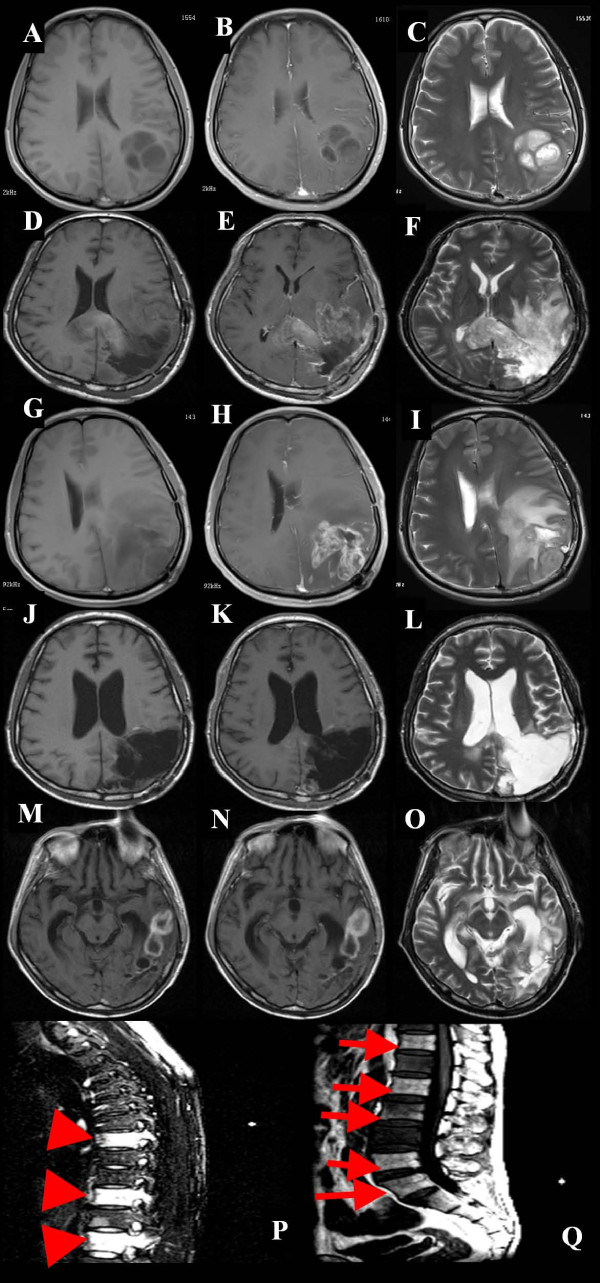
Representative axial MR images with gadolinium, taken on initial admission. (A) T_1_-weighted MR image (T_1_WI); (B) Contrast-enhanced T_1_WI and (C) T_2_-weighted MR image (T_2_WI) showing a left occipital tumor with mild contrast enhancing; (D) at one month follow up, T_1_WI; (E) contrast-enhanced T_1_WI and (F) T_2_WI showing no apparent enhanced lesion; (G) 8 months later after the first surgery, T_1_WI; (H) contrast-enhanced T_1_WI and (I) T_2_WI showing marked enhanced mass on the cavity and recurrence; (J) 48 h after the second surgery, T_1_WI; (K) contrast-enhanced T_1_WI, and (L) T_2_WI showing no apparent enhanced lesion; (M) 8 months later after combination radiotherapy and chemotherapy, T_1_WI; (N) contrast-enhanced T_1_WI and (O) T_2_WI showing marked enhanced mass of the wall in the posterior portion of the removal cavity and left temporal areas, and recurrence of the enhanced tumor. (P, Q) 8 months later after combination radiotherapy and chemotherapy, sagittal spinal contrast-enhanced T_1_WI after gadolinium infusion showing high-intensity mass lesion in (P) T7, T10, and T12 vertebral bodies (arrowheads) and (Q) T12, L2, L3, L5, and S1 vertebral bodies (arrows).
